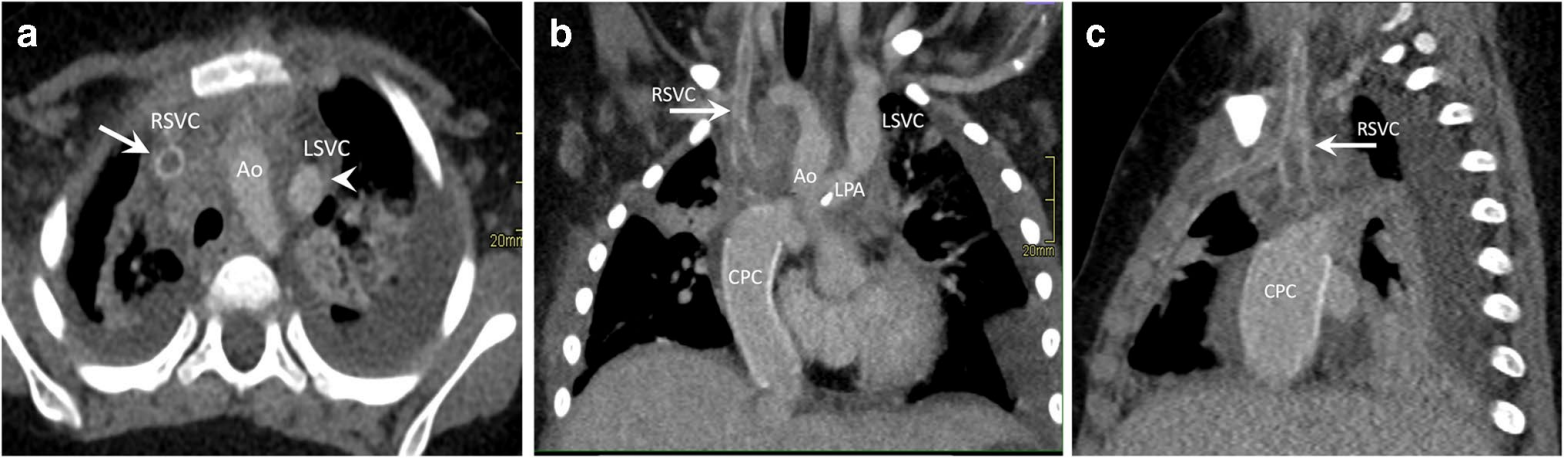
A 2-year-old girl, presenting with acute symptoms with low oxygen saturation 3 weeks after surgical repair to a total cavopulmonary connection. A right and left superior vena cava were anastomosed to the respective pulmonary arteries (bilateral Glenn procedure). Echocardiography did not reveal flow in the right superior vena cava. a–c Contrast-enhanced computed tomography with multiplanar reconstructions in axial (a) coronal (b) and sagittal (c) planes shows a patent left superior vena cava (SVC) with high density contrast in the lumen connected to the left pulmonary artery (*arrowhead* in a) and confirms the presence of an occluding thrombosis with low density and rim enhancement in the right SVC (*arrow in*
